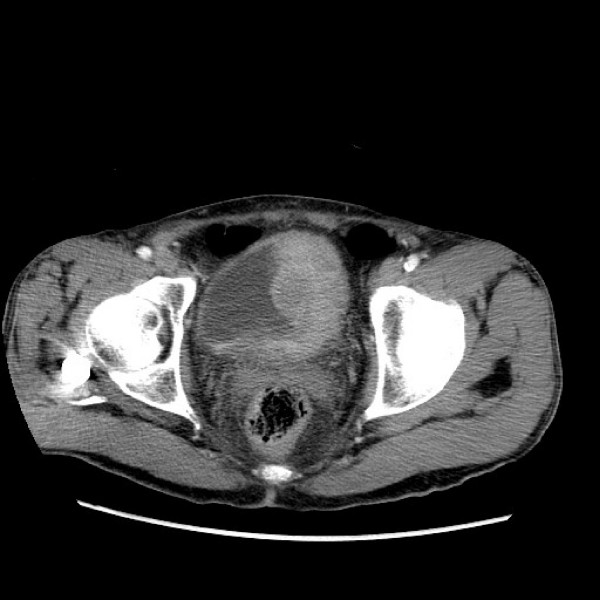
CT scan of Case 1. large lobulated, enhancing soft tissue mass affecting the left side of the bladder (a).
